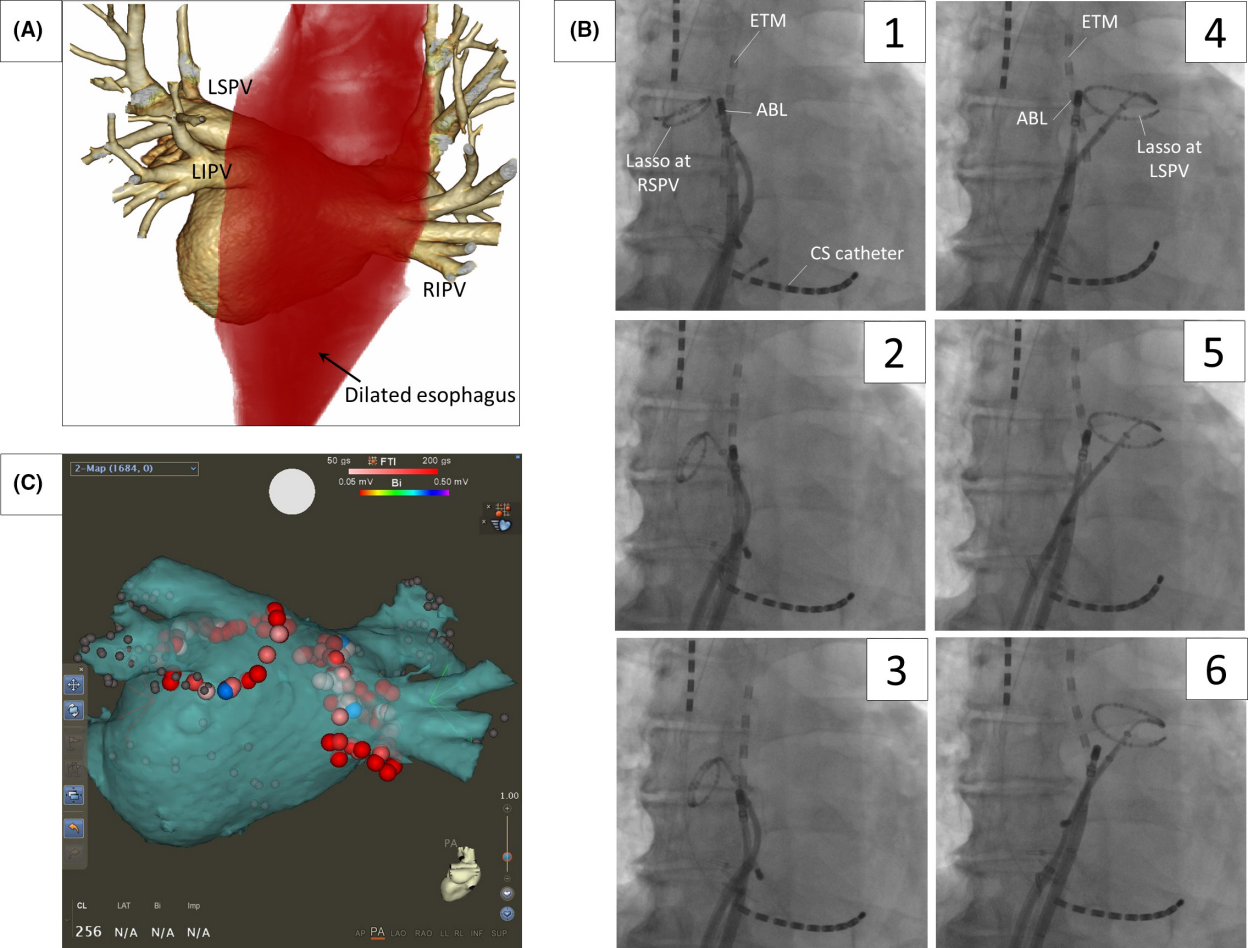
(A) Three‐dimensional computed tomography revealed a dilated esophagus facing the entire posterior wall of the LA. The red shadow indicates the dilated esophagus. (B) The Esophastar^®^ was tentatively placed at the right (1–3) and left (4–6) posterior ablation sites. (C) The EEPVI was successfully performed using CARTO3. The tags indicate the ablation points for the EEPVI. LSPV, left superior pulmonary vein; LIPV, left inferior pulmonary vein; RIPV, right inferior pulmonary vein; ETM, esophageal temperature monitor; ABL, ablation catheter; CS, coronary sinus; EEPVI, extensive encircling pulmonary vein isolation.
